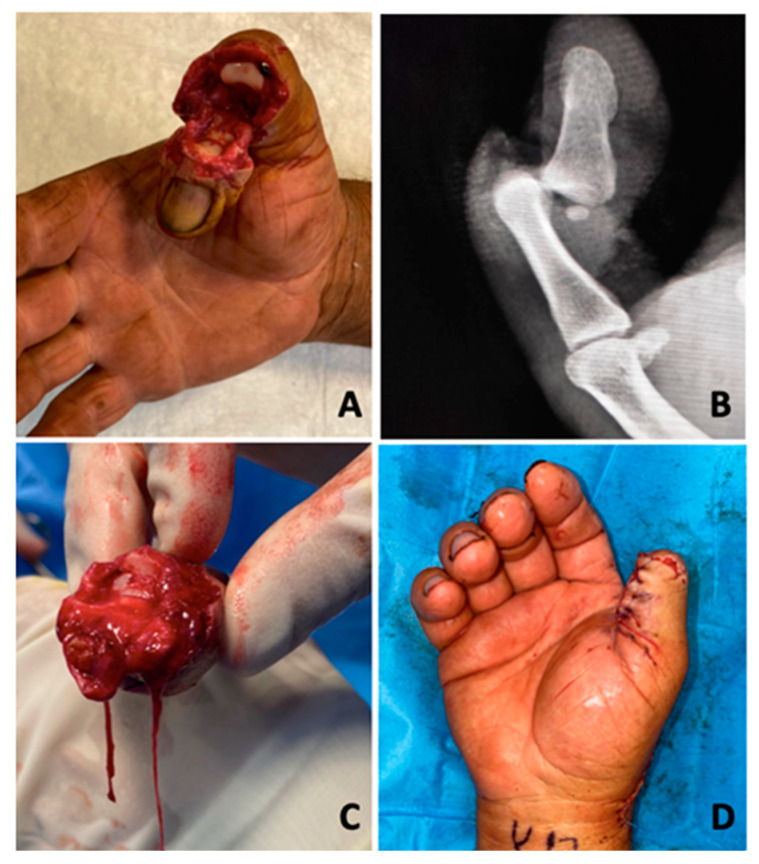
Avulsion injury of the distal phalanx of the thumb. (A) Clinical presentation as subamputation, tissue continuity is represented only by the flexor longus pollicis; (B) Radiograph shows volar dislocation of the distal phalanx without fracture; (C) Clinical image of the extension pedicle after; (D) Final aspect after regularisation. Final aspect after reduction.
